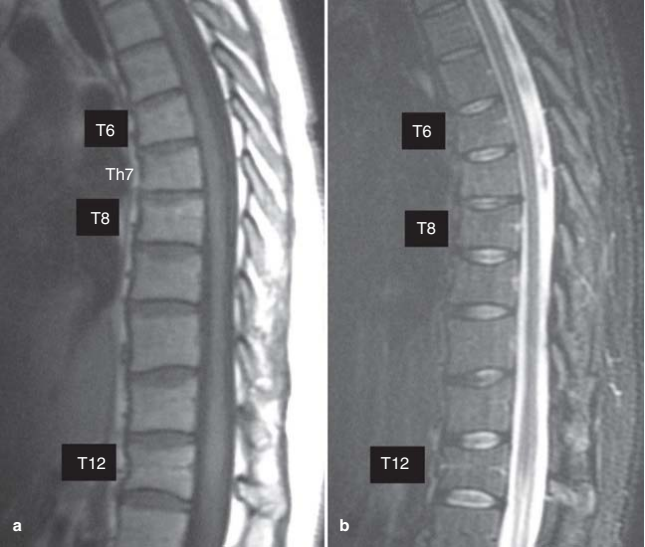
Magnetic resonance imaging (MRI) of the vertebrae in case 4 at 7 months after prescribing vitamin K_2_. MRI showed no signal intensities on T1- (A) and T2- (B) weighted images.
